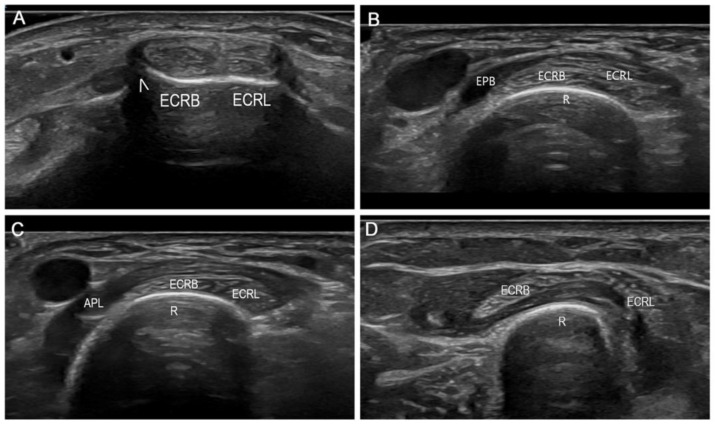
Ultrasound scanning of the second dorsal compartment tendons and the first dorsal compartment muscles, short-axis view. (A) Extensor carpi radialis brevis (ECRB) and extensor carpi radialis longus (ECRL) at distal dorsal tubercle of the radius level (arrowhead). (B) Crossing between the second dorsal compartment tendons (ECRB, ECRL) and the extensor pollicis brevis (EPB) muscle. R, radius. (C) Crossing between the second dorsal compartment tendons (ECRB, ECRL) and the abductor pollicis longus (APL) muscle. R, radius. (D) Musculotendinous junction of the ECRB and ECRL. R, radius.
